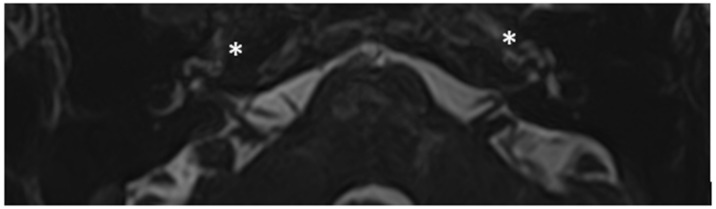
MR images of patient #4 with otosclerosis. MR cisternography, axial plane. Note the fluid signal in the newly formed cavity (white asterisks).
